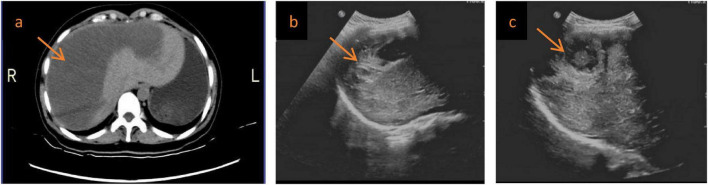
Two-month follow-up imaging after embolization. (a) Follow-up abdominal CT at 2 months showed marked reduction in the size of the right-lobe subcapsular hematoma compared with admission (arrow), with lower attenuation and less capsular bulging, without imaging evidence of recurrent active bleeding. (b,c) Hepatic ultrasonography at the same time point demonstrated residual strip-like and arcuate mildly hyperechoic subcapsular areas in the right hepatic lobe (arrow), consistent with organizing and resorbing hematoma. The surrounding hepatic parenchyma appeared homogeneous, and no obvious intraperitoneal free fluid was detected.
